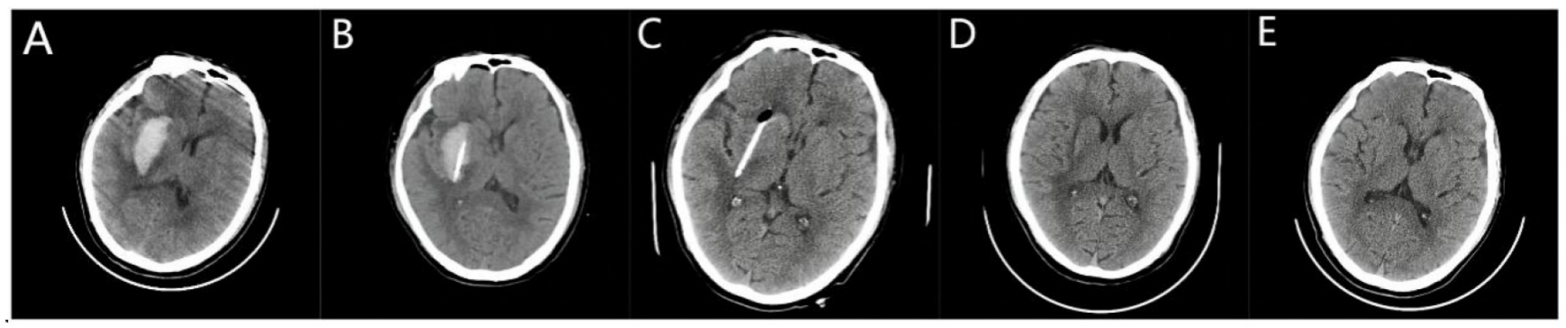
Stereotactic catheterization through the long axis of hematoma for basal ganglia hemorrhage. (A) A preoperative head Computed Tomography (CT) showed that the bleeding volume was ~29 ml. (B) A three-dimensional CT performed 6 h after surgery confirmed the position of the drainage tube along the long axis of the hematoma, with a hematoma volume of ~20 ml. (C) Following the injection of urokinase, the hematoma was completely drained by the third day after surgery. The high-density area indicates the drainage tube with a hematoma volume of 0 ml. (D) The bleeding site 7 days after surgery was slightly softer. (E) On the 20th day post-surgery, the softening lesion at the bleeding site was reduced.
